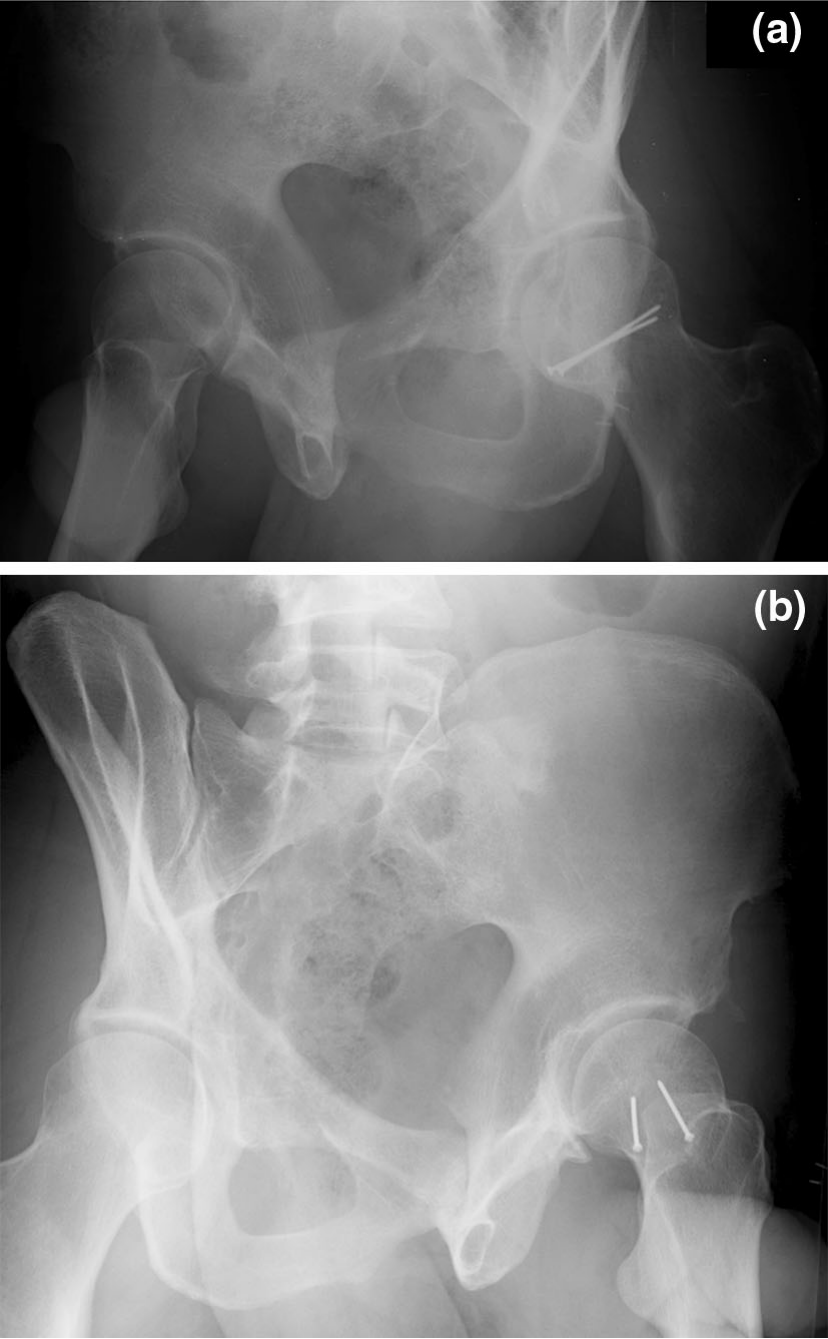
a Postoperative obturator and b iliac oblique radiographs of patient shown in Fig. 1, demonstrating fixation of the femoral head fracture with two 2.0 mm minifragment screws
